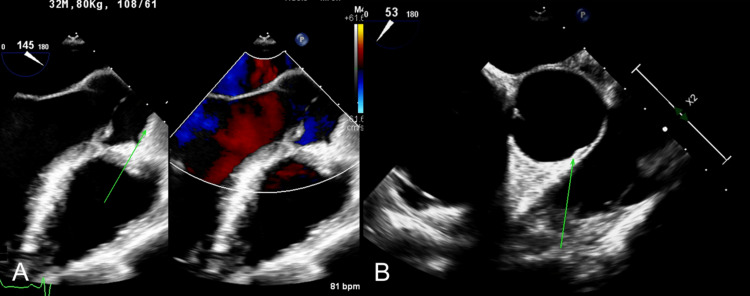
TEE mid-esophageal long axis view with and without Doppler (A) and ascending aorta short axis view (B) showing mild thickening of the ascending aortic wall (green arrow), suggestive of aortitis. TEE: transesophageal echocardiogram
